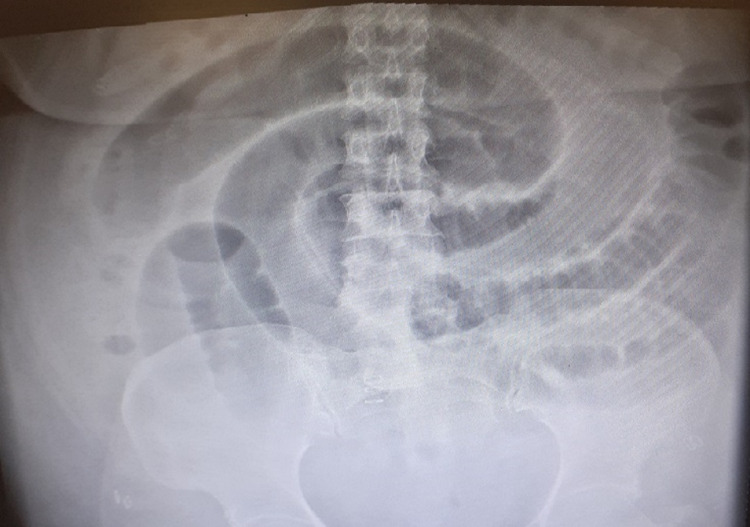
POD 4 X-ray of the abdomen showing dilated small bowel loops POD: postoperative day
